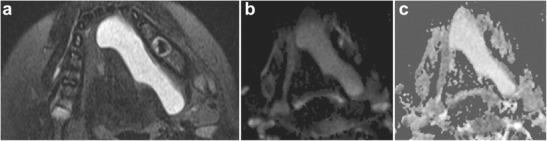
Sublingual space ranula. a Axial T2-weighted MR image shows a high-signal lesion within the left sublingual space. b Axial diffusion-weighted image and c ADC map demonstrates lack of restricted diffusion, confirming the diagnosis (as opposed to an epidermoid, which would have restricted diffusion)
